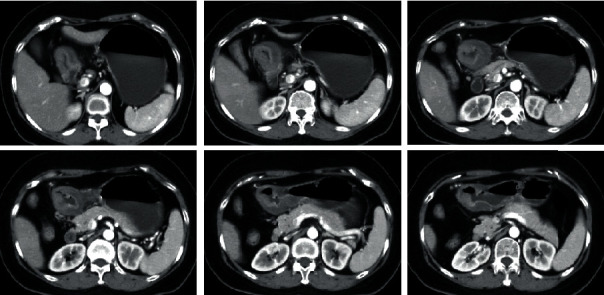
CT images of lymphoma (female patient, 67 years old).
